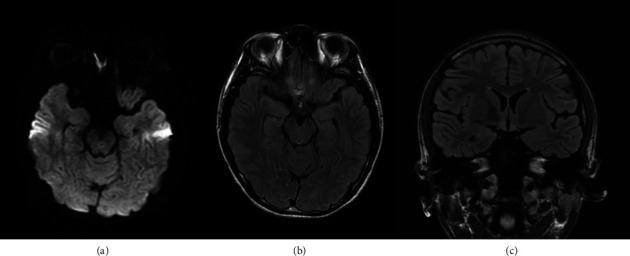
Normal magnetic resonance imaging (MRI) findings in the mesial temporal lobe on Day 30 after symptom onset. (a) Axial diffusion-weighted imaging (DWI). (b) Axial fluid-attenuated inversion recovery (FLAIR) imaging. (c) Coronal FLAIR imaging.
